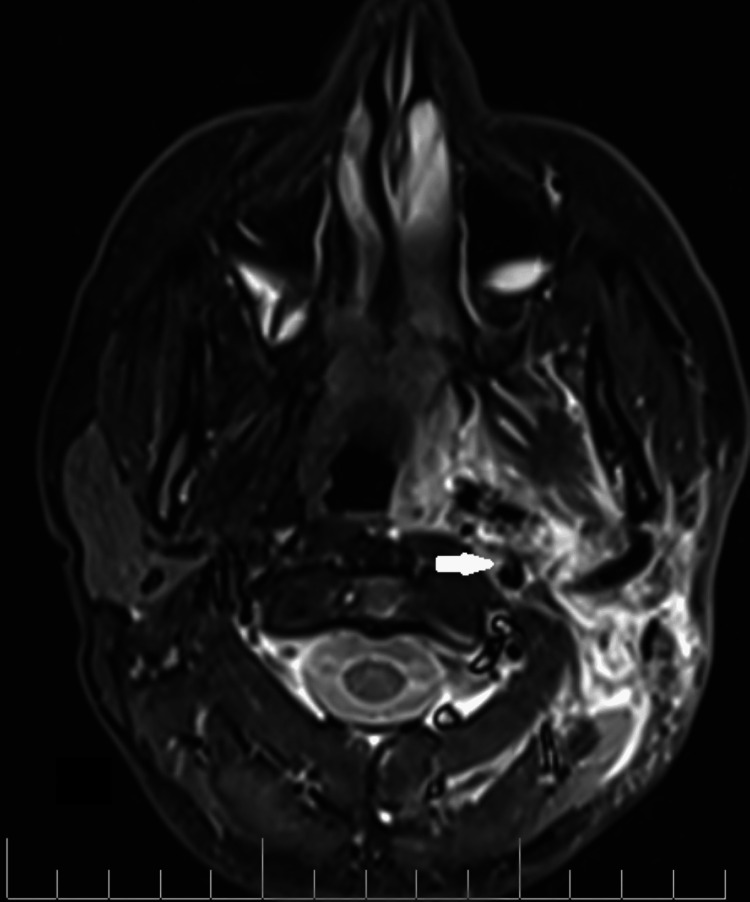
Postoperative MRI showing the parapharyngeal space (white arrow) with postoperative changes and no signs of tumor
